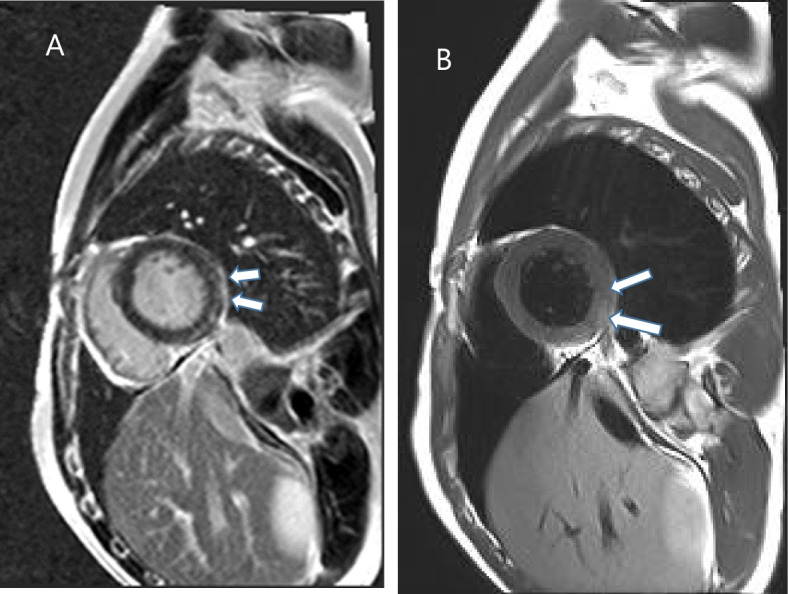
Cardiac magnetic resonance image. Short-axis late gadolinium enhanced (LGE) MR images (a) show subepicardial enhancement (arrow) in the anterolateral and inferolateral wall of mid base left ventricle (LV) level. Short-axis T2-weighted MR images (b) show high signal intensities (arrows) in those regions with enhancement regions, suggesting myocardial edema
